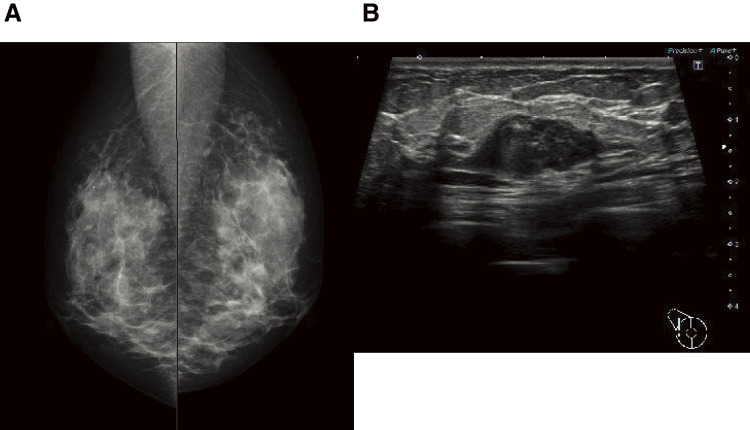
(A) Mammography showed no tumor or calcification in her bilateral breast. (B) Ultrasonography showed a circumscribed, irregular hypoechoic mass in her right breast.
